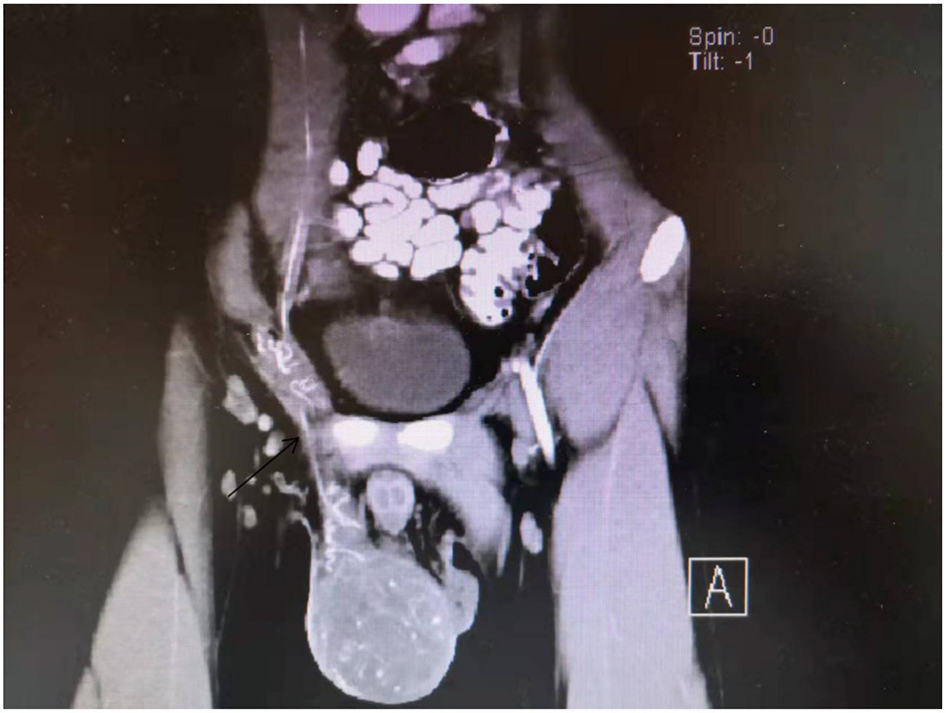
Enhanced CT scan show that the spermatic vessels.
